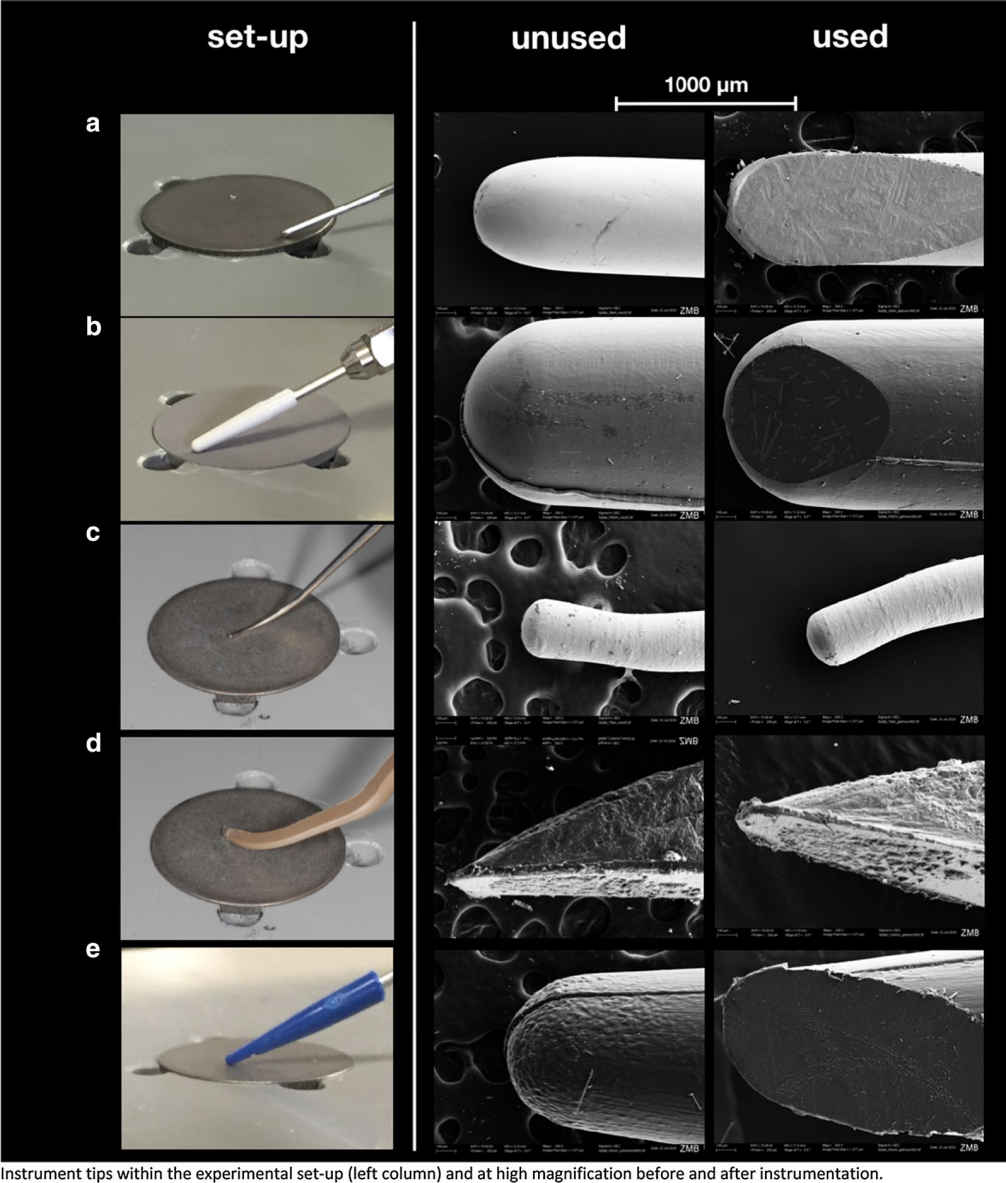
Ultrasonic tips in the set-up, before use and after use. Instrument tips within the experimental set-up (left column) and at high magnification before and after instrumentation. a steel („used“ after 40 × 3 cycles), b PEEK („used“ after 2 × 3 cycles), c titanium („used“ after 4 × 4 cycles), d carbon(„used“ after 2 × 3 cycles), e resin („used“ after 1 × 3 cycles)
